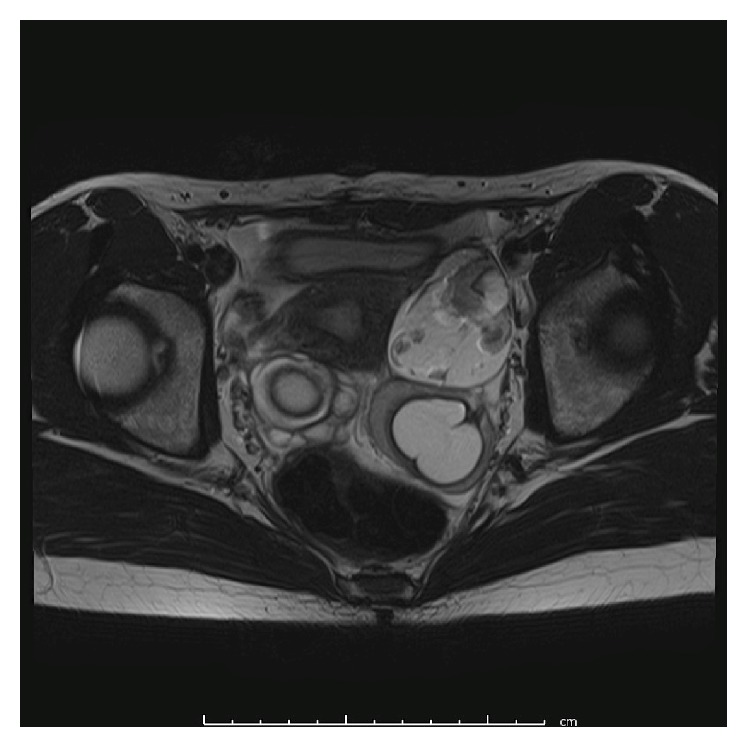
Pelvic magnetic resonance imaging (T2-weighted image) before the second surgery of Case  2. Bilateral ovarian tumors are detected (right side: 4.4 cm, left side: 8.0 cm in diameter).
